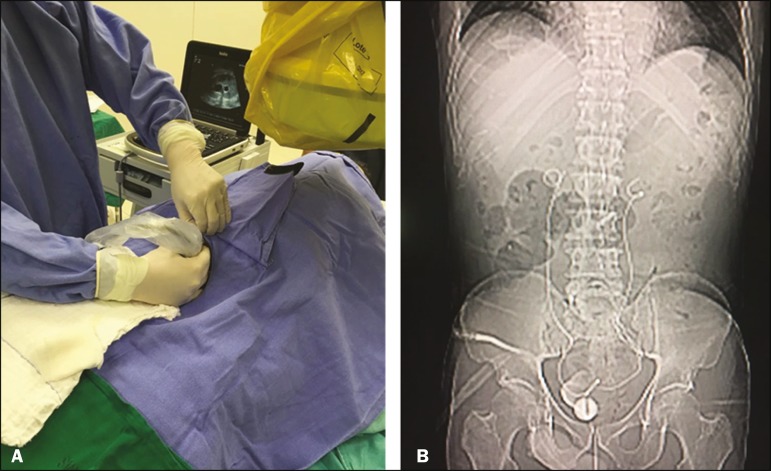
Planning of percutaneous JJ stenting with ultrasound of the urinary tract
